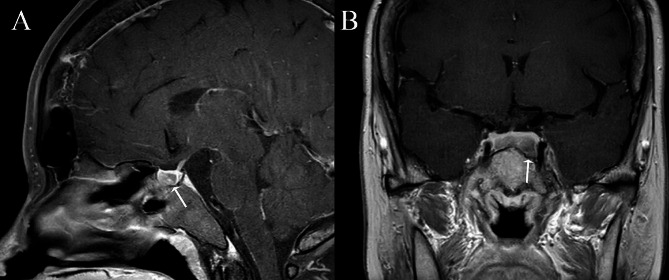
MRI image of the pituitary tumor. A, T1-weighted image (T1-WI) contrast-enhanced sagittal plane reveals a hypointense nodule, B, T1-WI contrast-enhanced coronal plane shows a macroadenoma across the right and left of the pituitary, without extension into the cavernous sinus or optical chiasm. Arrows indicated the location of the lesion
